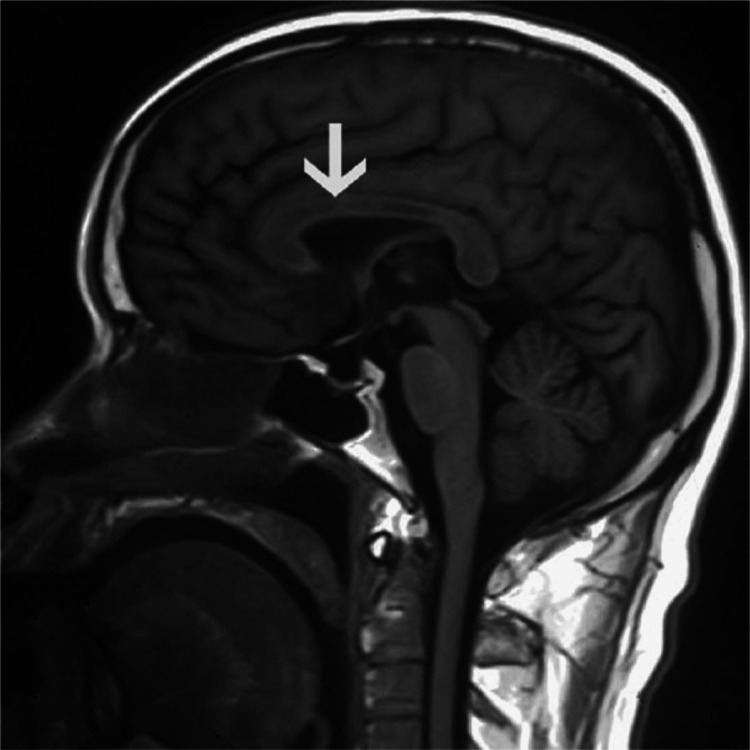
MRI T1-weighted sagittal view showing the sandwich sign - hypointense center of corpus callosum but normal area around it. Reference [30] is licensed under CC BY-NC 4.0
